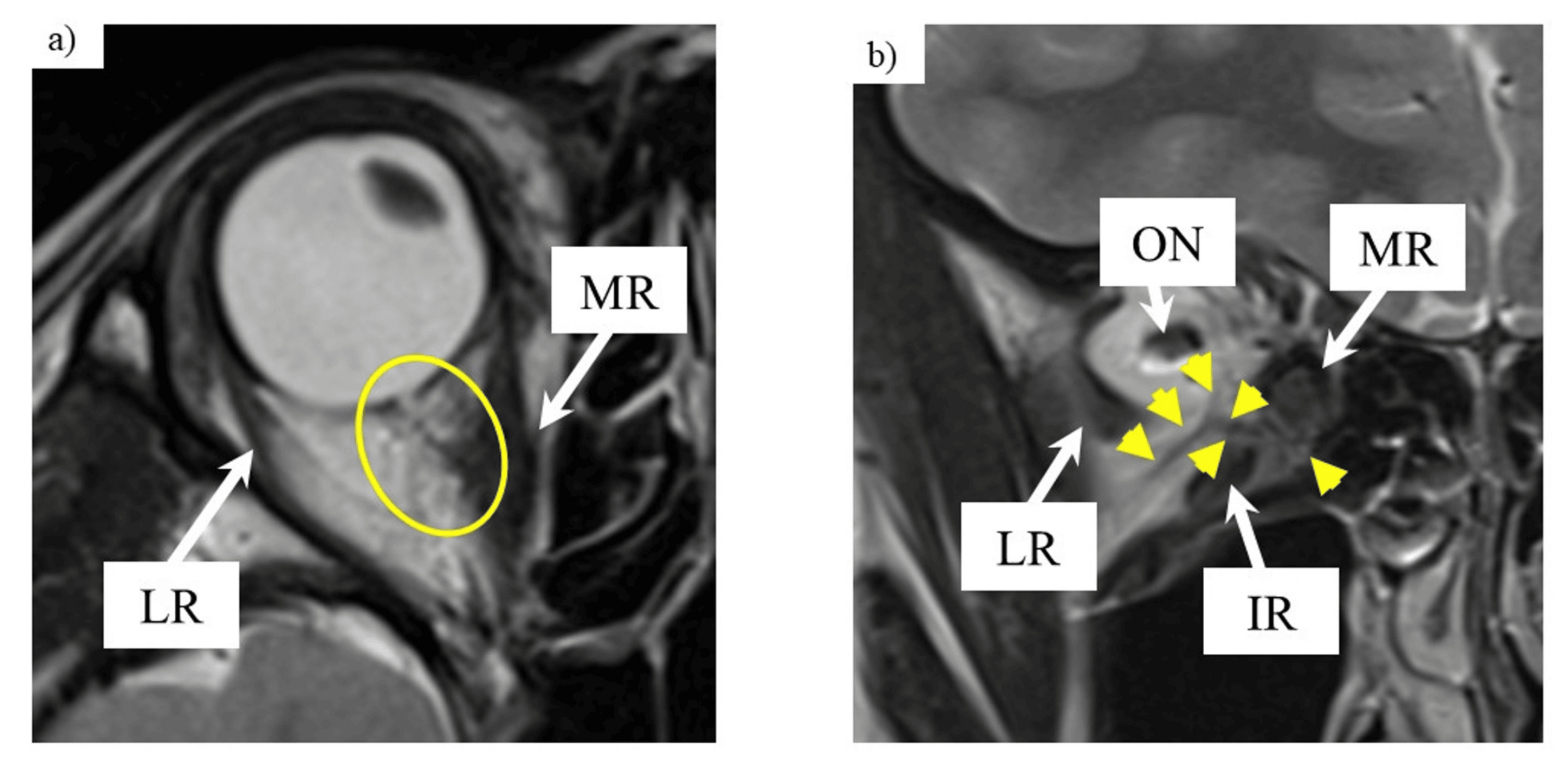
MRI of the right orbit obtained at 12 years of age (a) Axial T2-weighted MRI. An accessory extraocular muscle is seen extending from the right MR to the posterior orbit (yellow circle). (b) Coronal T2-weighted MRI. A diffuse abnormal structure is visible between the MR and IR, with signal intensity intermediate between adipose tissue and extraocular muscle (yellow arrows). This tissue was identified as an accessory extraocular muscle. All images are 3.0-mm thick. LR: lateral rectus muscle, ON: optic nerve, MR: medial rectus muscle, IR: inferior rectus muscle, MRI: magnetic resonance imaging
